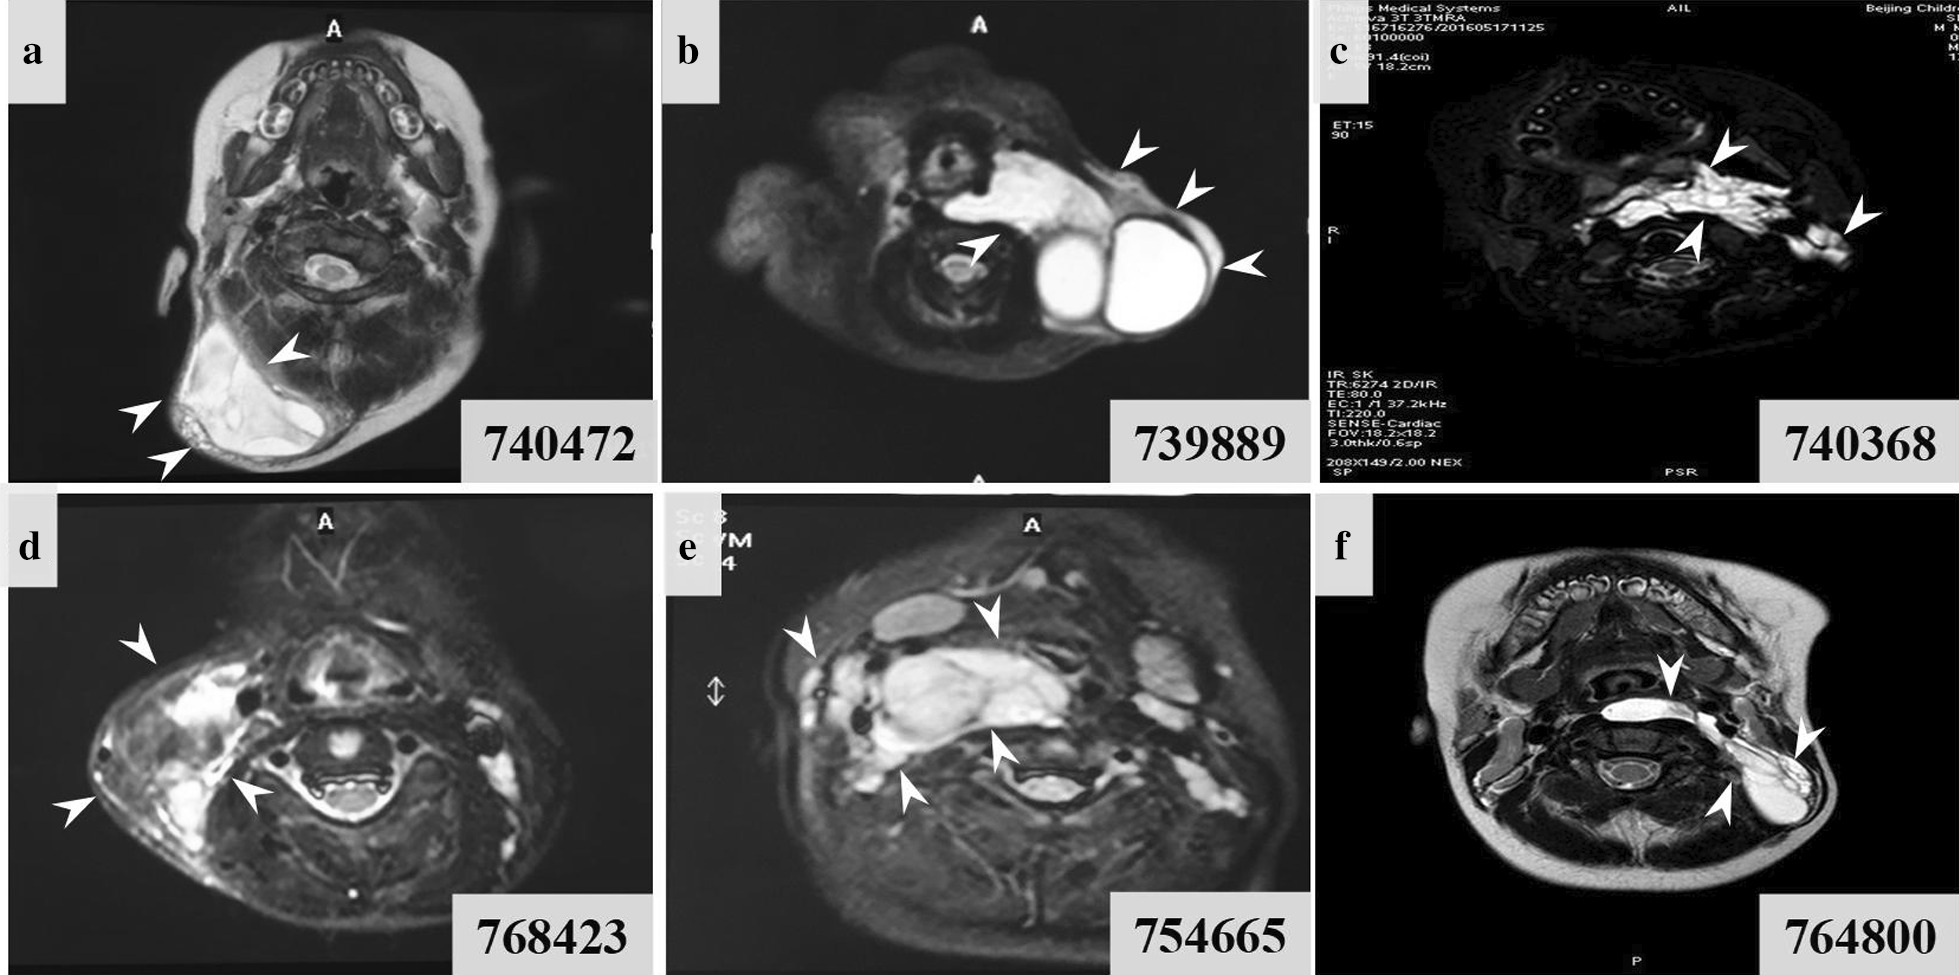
MRI image of each subtype of LMs. a, b MRI (T2 image in axial view) reveal macro-cystic lesions (more than 2 cm in diameter) in head and neck region. c MRI (T2 image in axial view) reveals micro-cystic lesion in parapharyngeal spaces, retropharyngeal space, and prevertebral space. d The axial postgadolinium contrast enhanced T1-weighted MRI LM with microcysts, with enhancing setae between the individual cysts. e, f MRI (T2 image in axial view) reveal macrocysts and microcysts
